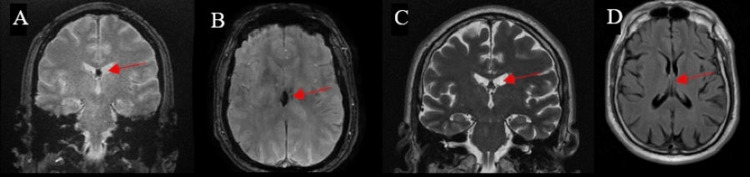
Brain MRI during hospitalization A: Brain MRI GRE coronal slice with fornix hypointensity (arrow). B: MRI GRE axial slice with fornix hypointensity (arrow). C: Non-contrast MRI T2 coronal slice with fornix hypointensity (arrow). C: Contrasted MRI T2 FLAIR axial slice of the fornix demonstrating no signs of mass effect or edema (arrow). MRI: magnetic resonance imaging, GRE: gradient echo sequence, FLAIR: fluid-attenuated inversion recovery
